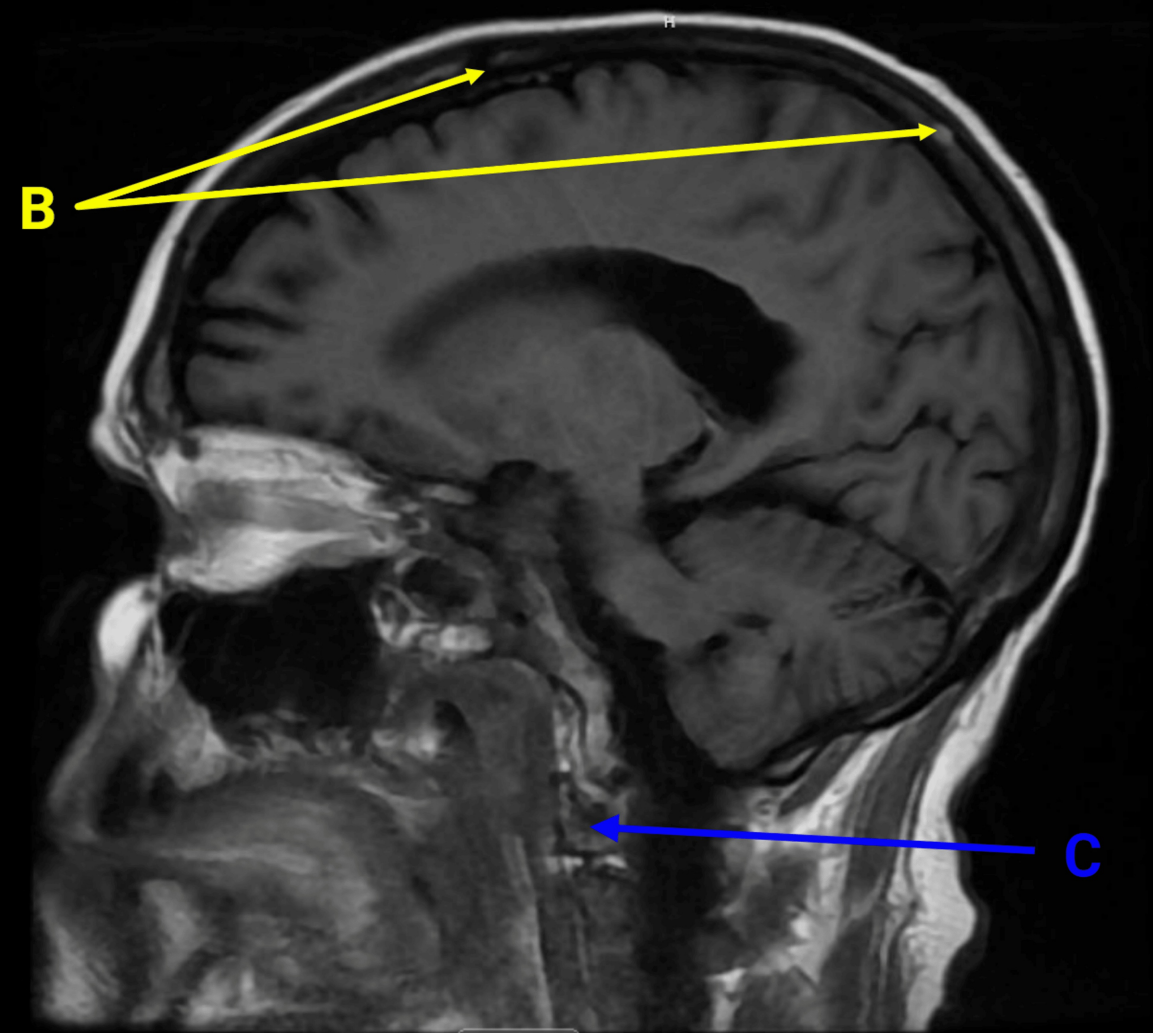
Magnetic resonance imaging (MRI) of the brain with intravenous contrast B: Patchy areas of abnormal T1 signal throughout the calvarium (highlighted in yellow), consistent with new osseous metastases. C: Similar patchy areas of abnormal T1 signal in the upper cervical spine (highlighted in blue), also indicative of osseous metastases.
